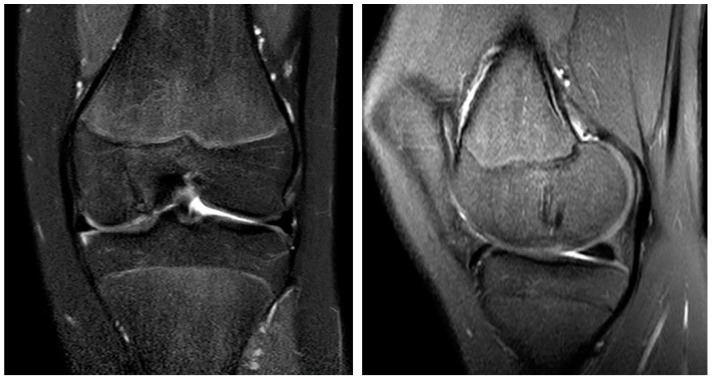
The MRI 10 months after surgery shows the almost entirely healed bone and the continuing remodeling process of the magnesium screws in the coronar (left) and sagittal plane (right).
